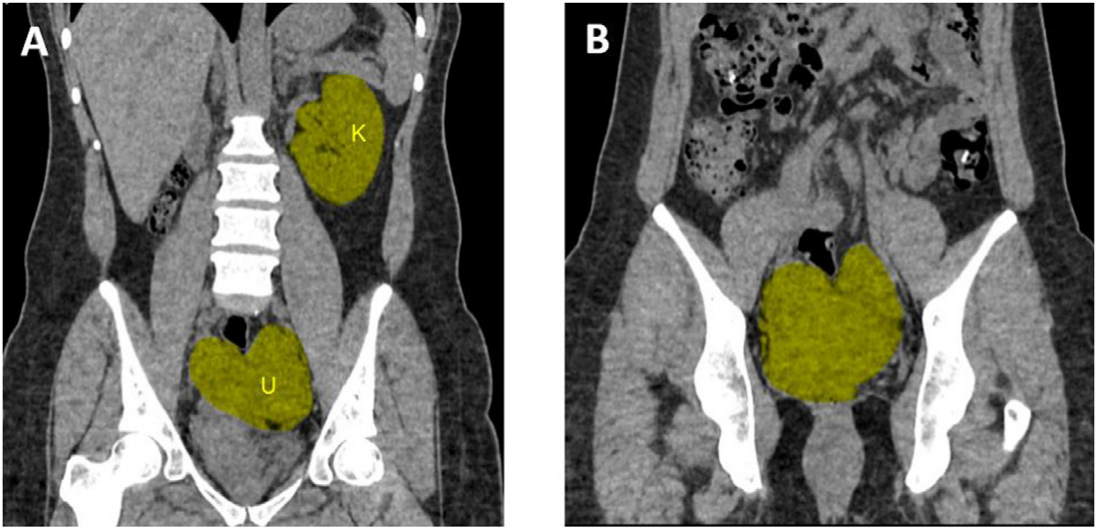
Coronal CT scan of the abdomen and pelvis showing (A) Müllerian anomaly as a bicornuate uterus (U) and absence of the right kidney—highlighted areas, (B) bicornuate uterus (highlighted area).
